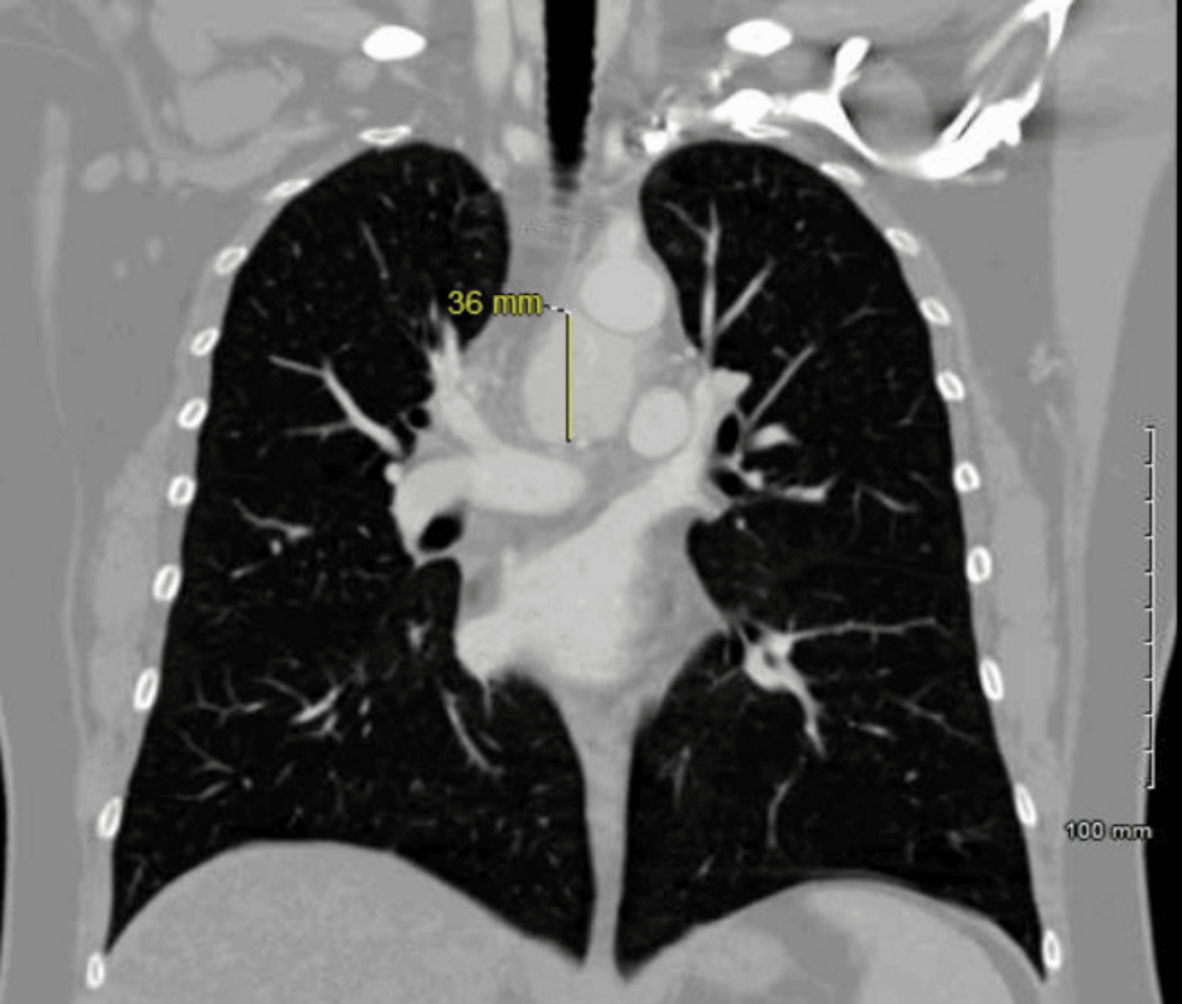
Coronal view from CT chest with contrast showing a hyper-enhancing mediastinal mass
